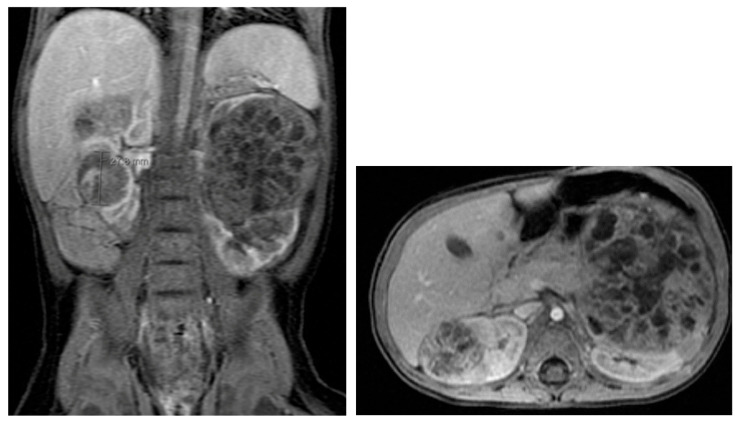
MRI shows bilateral nephroblastomatosis with bilateral nephroblastoma in a 2-year-old female.
